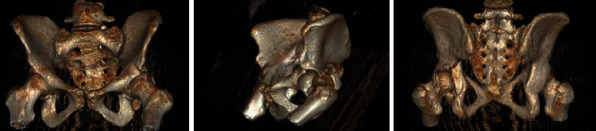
Three-dimensional reconstructions of the CT scan obtained in the initial trauma evaluation, further delineating the fracture pattern.
